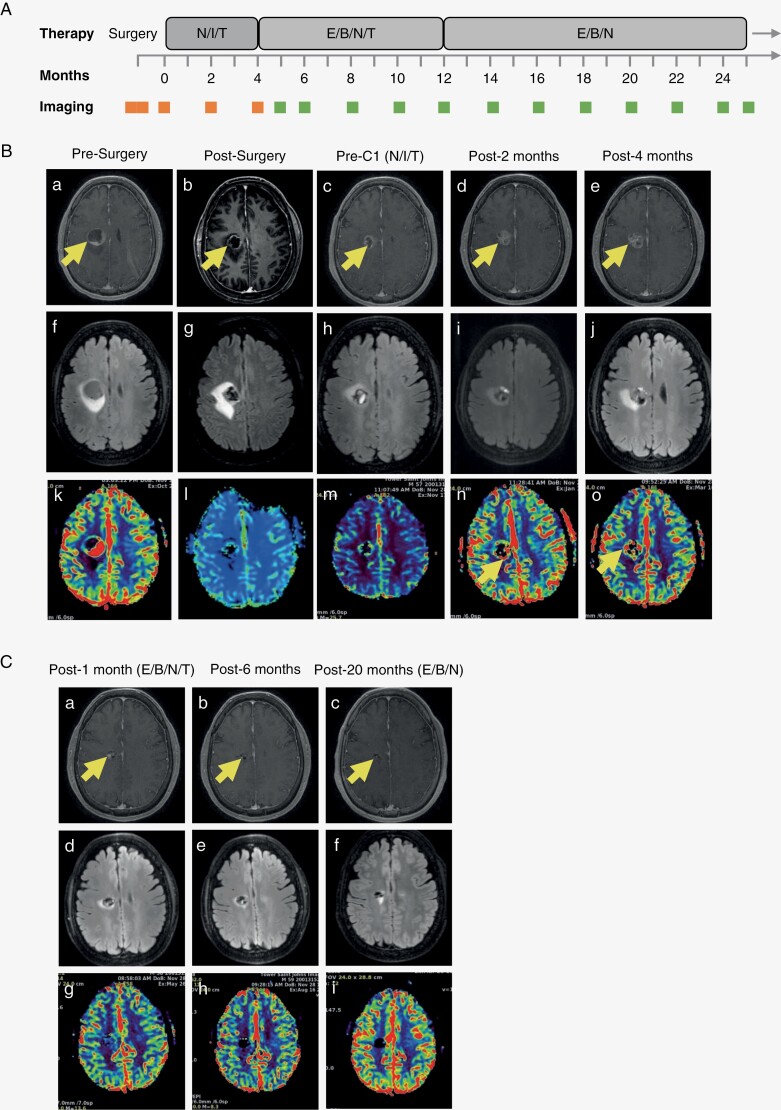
Treatment protocol and radiological findings. (A) Timeline and treatment protocol, with rectangles indicating times of brain scans. The patient was treated with nivolumab 240 mg every 2 weeks, ipilimumab 1 m/kg every 6 weeks, and temozolomide (N/I/T) and followed with monthly scans. At progression, ipilimumab was discontinued, and encorafenib and binimetinib were added to the treatment regimen and (E/B/N/T). Temozolomide was discontinued after six cycles combined with E/B/N. (B). T1 postcontrast images (a–e), edema on axial FLAIR sequences (f–j), and axial T1 perfusion sequences (k–o) over time from initial surgery to progression on N/I/T. (C) T1 postcontrast (a–c), edema on axial FLAIR sequences (d–e) and axial T1 perfusion sequences (g–i) with E/B/N/T.
